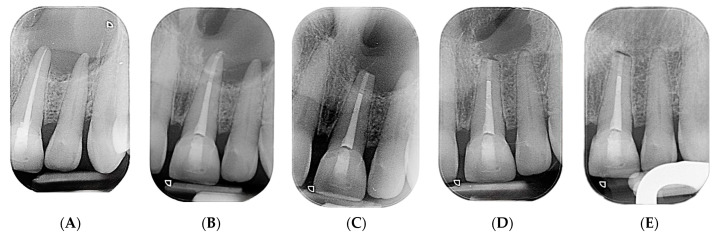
(A) Periapical radiograph taken before endodontic treatment, (B) periapical radiograph taken after nonsurgical root canal treatment, (C) immediate postoperative periapical radiograph following apical surgery, (D) one-year postoperative periapical radiograph, and (E) periapical radiograph from 30 months post operation.
